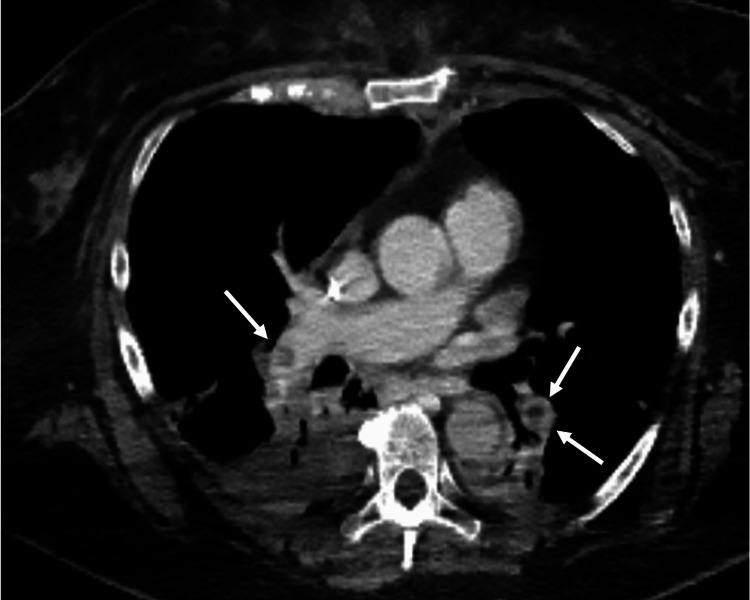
Chest CT scan following ECMO initiation. Axial contrast-enhanced CT image of the chest confirmed bilateral pulmonary embolisms in the right pulmonary artery and in the lobar artery of the left lower lobe (white arrows). ECMO = extracorporeal membrane oxygenation
